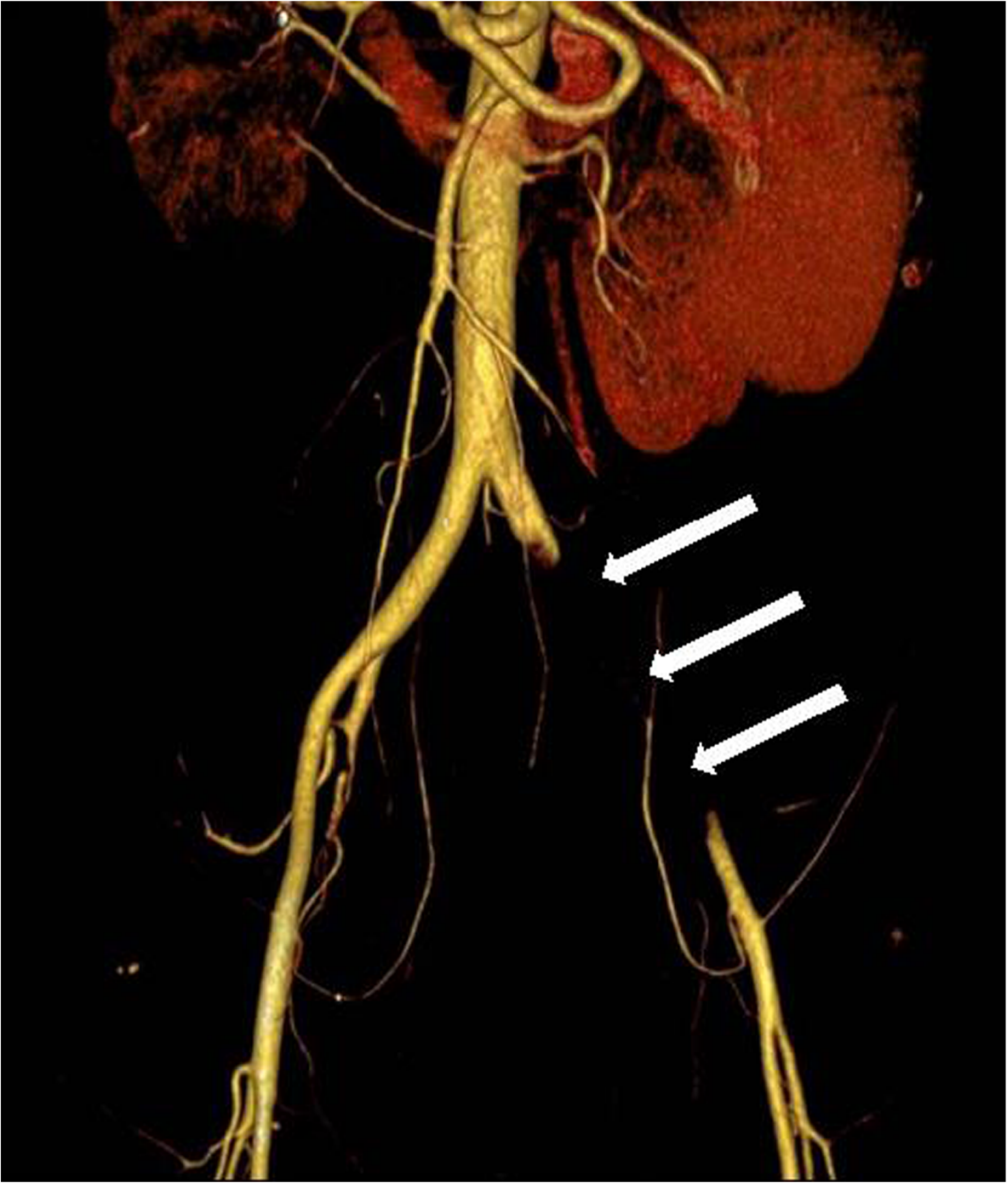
Occlusion of the left common and internal iliac artery (*arrows*) is detected by CT-angiography
